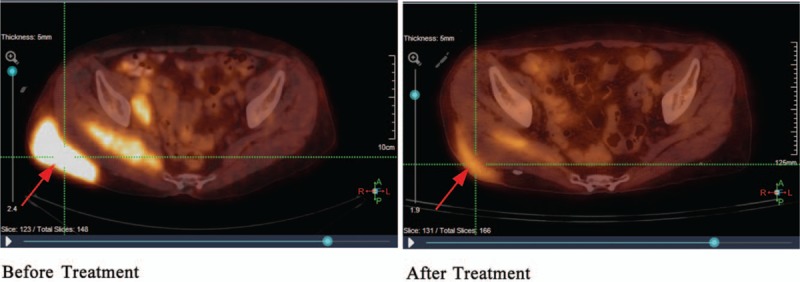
Comparison of the PET/CT results before and after treatment. PET/CT = positron emission tomography/computed tomography.
